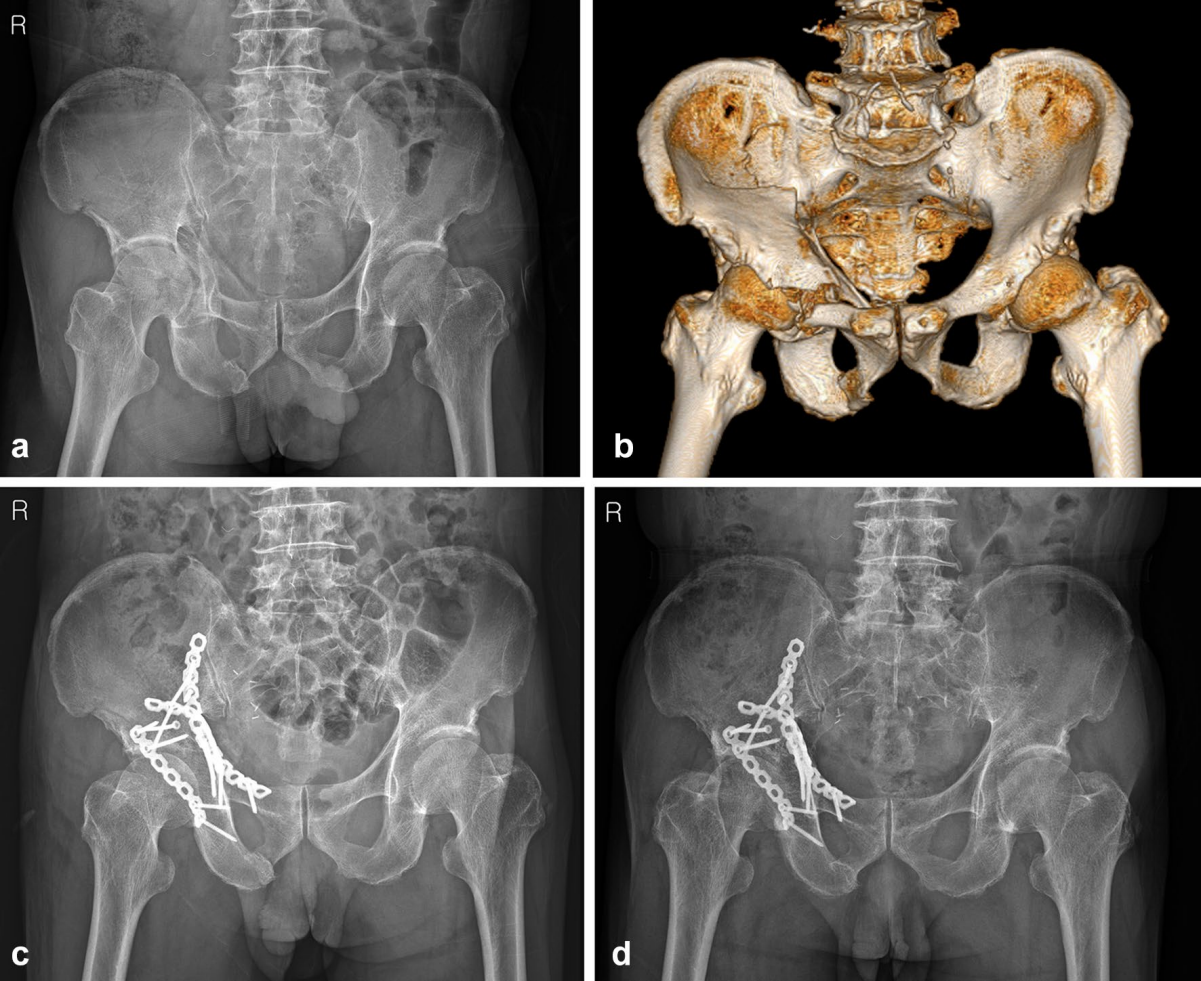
(a) pelvis anteroposterior radiograph and (b) 3D-CT of a 61-year-old male, superomedially displaced acetabular fracture with anterior wall fragment. (c) Immediate postoperative and (d) 77 months after surgery radiograph, after fixed with conventional reconstruction plate and additional buttress plate through modified ilioinguinal approach.
